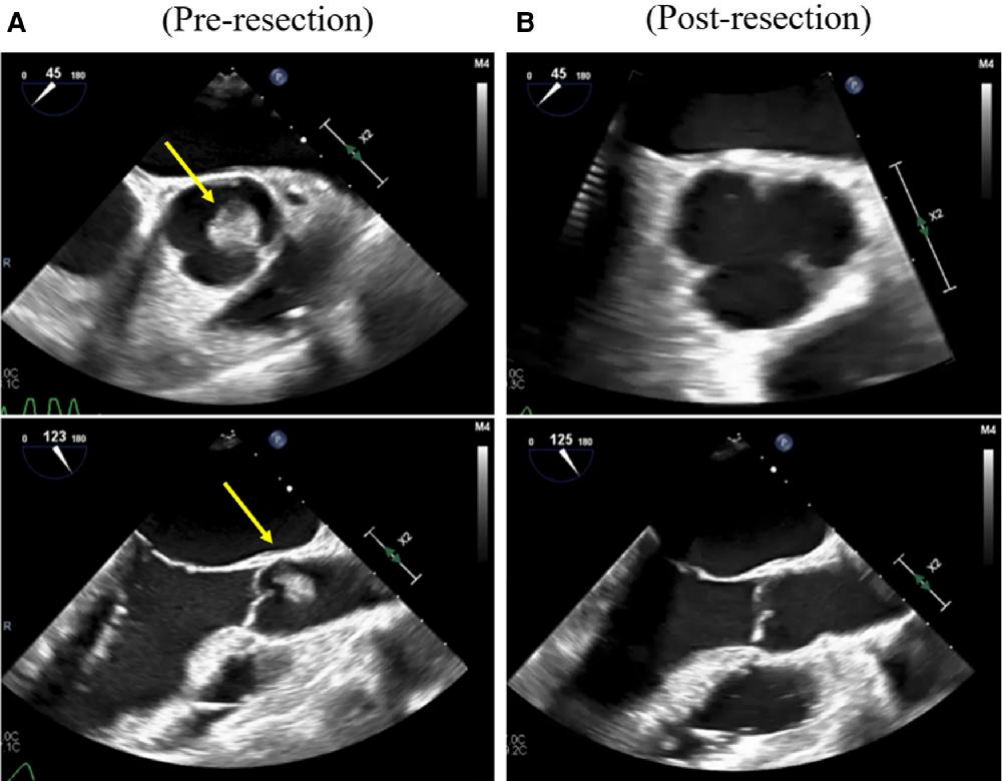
Intraoperative transoesophageal echocardiography (TEE) findings. (*A*) Intraoperative TEE identifying a soft tissue mass attached to the left coronary cusp of the aortic valve (arrow). (*B*) Postoperative TEE confirming successful tumour resection with preserved aortic valve function.
